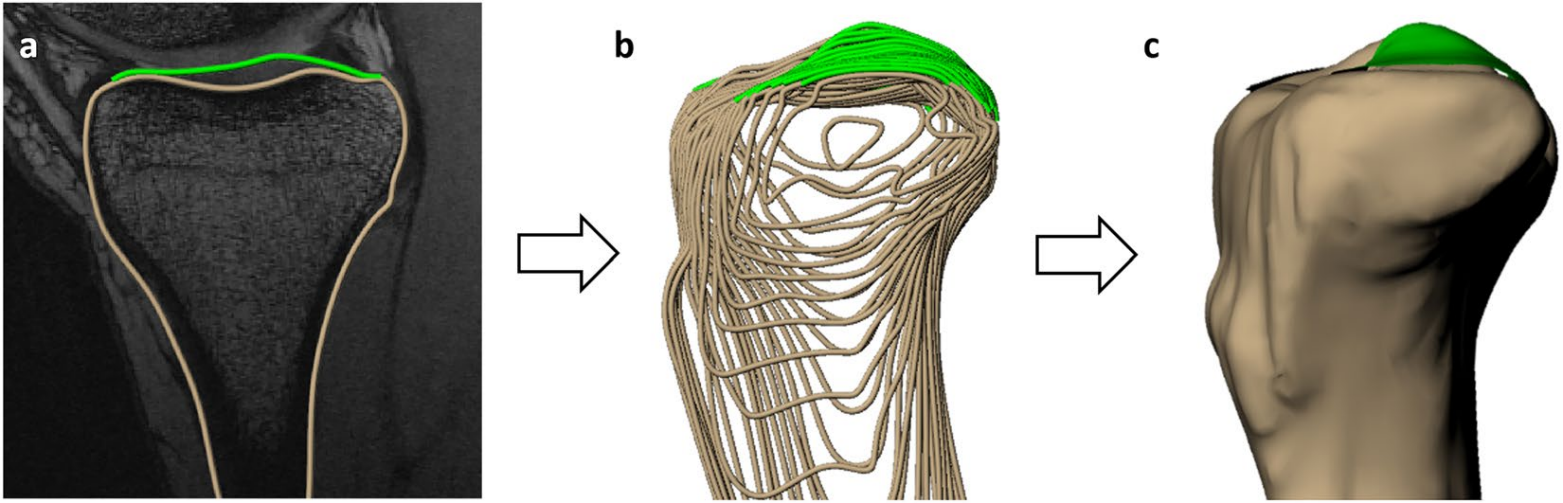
3D joint model creation from MRI (sagittal view, left knee), showing a) segmentation of articular cartilage and bone in a single MRI slice, b) stacked wireframe model of segmented surfaces, and c) 3D surface mesh model of the tibia and tibial cartilage.
